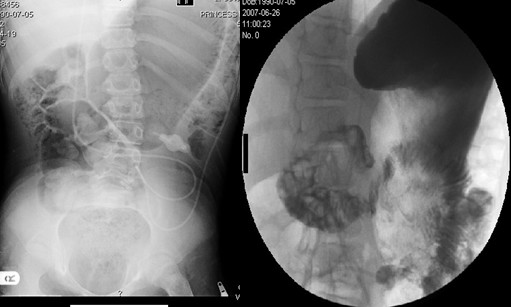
Plain abdomen X-ray and gastrografin studies.
